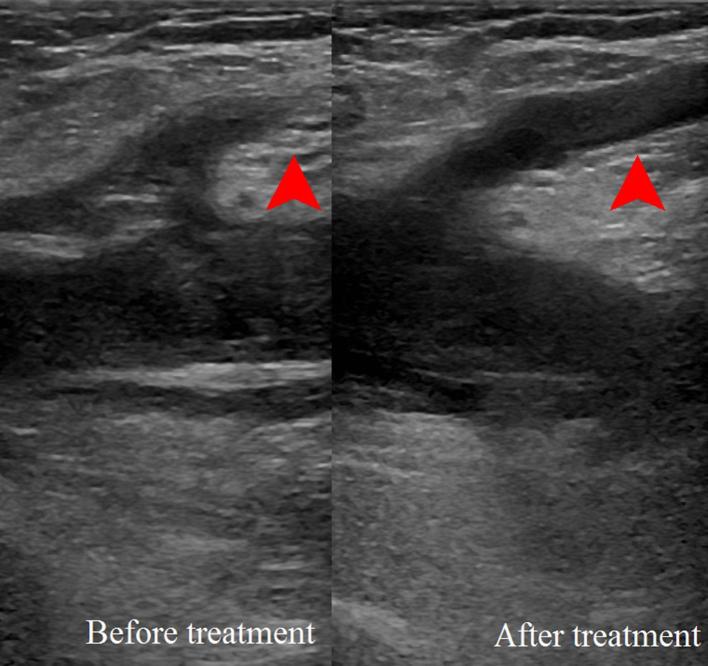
Ultrasound scan showing improvement in the right saphenofemoral
